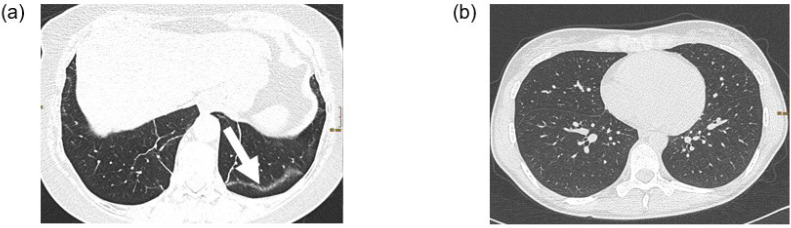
Two examples of chest CT scans. (a) CT examination in supine position of a 62 year old female patient with post-COVID-19 syndrome. The lung parenchyma shows subpleural bands (arrow) in the left lower lobe as sign of residual post-COVID changes. (b) CT examination in prone position of a 32 year old female patient with post-COVID-19 syndrome. The lung parenchyma is unremarkable.
